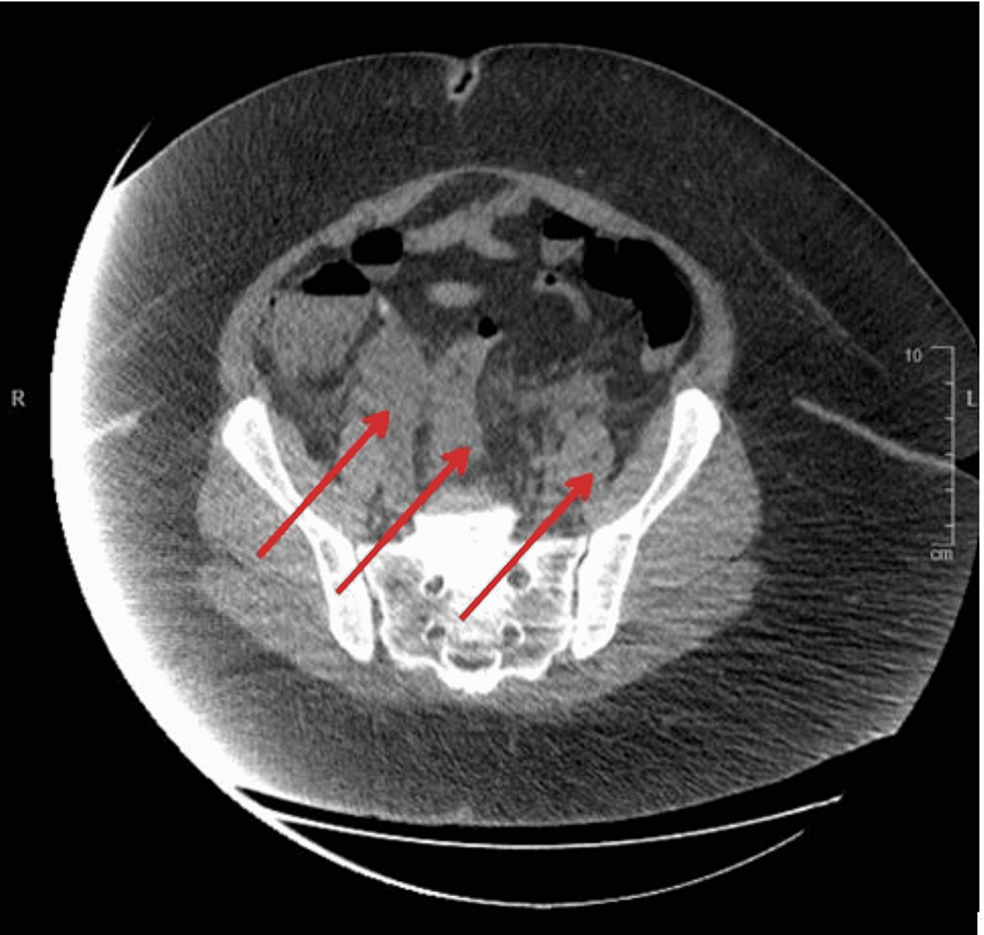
Abdominal CT showing undetermined pelvic fluid (indicated by red arrows)
